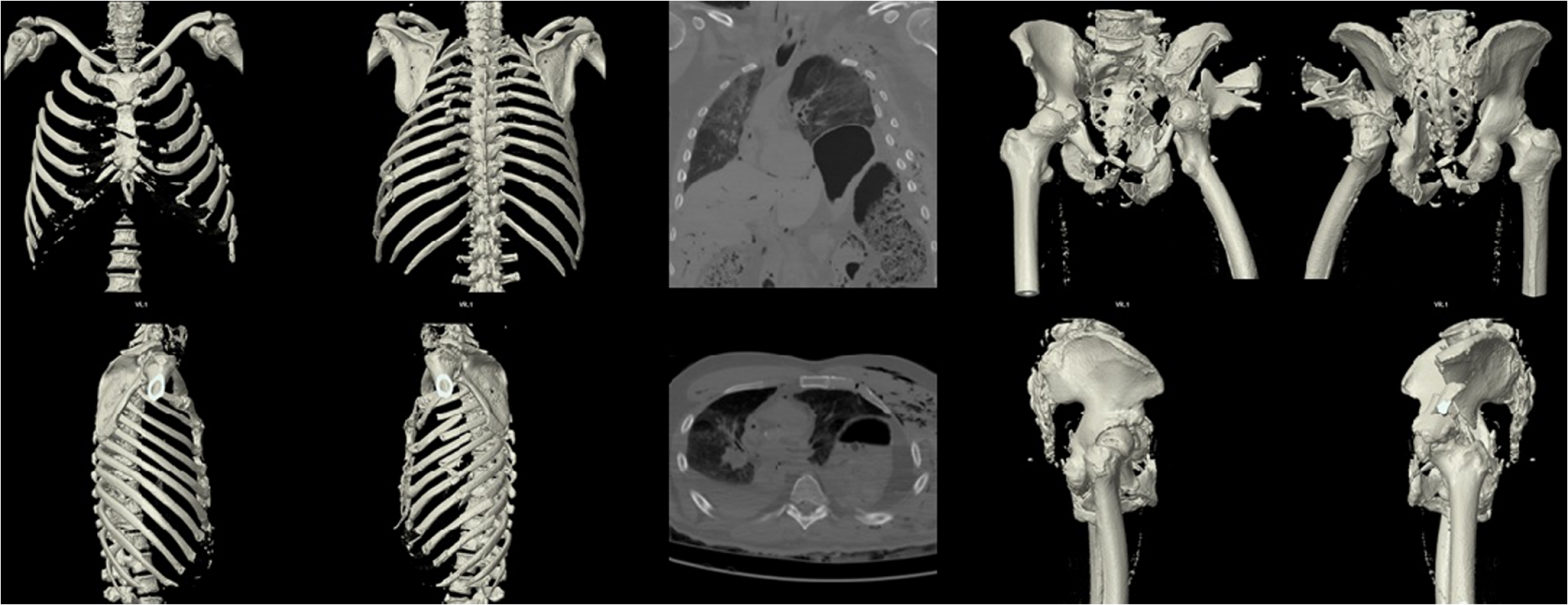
Example of the injury patterns of the deceased obtained from the post-mortem computed tomography data showing severe chest and pelvic trauma after truck rollover/impact trauma
